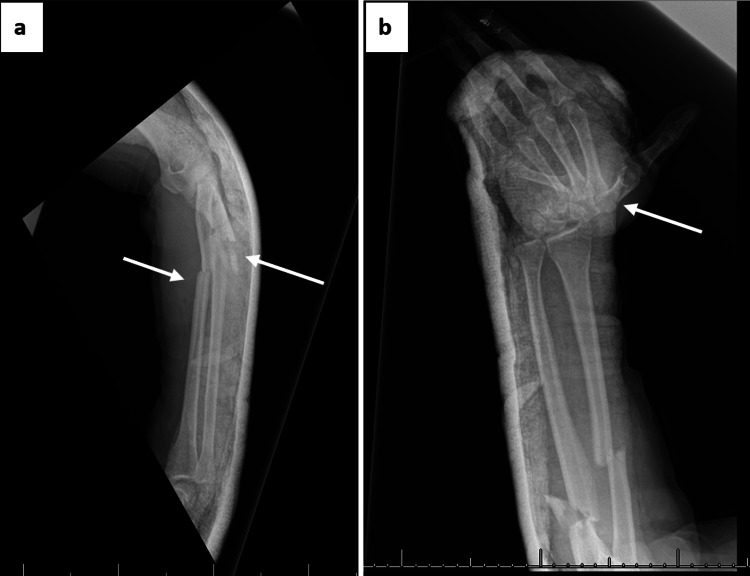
Initial radiographs: (a) lateral view showing comminuted fractures of the radius and ulna, (b) anteroposterior view demonstrating ulnar styloid fracture and wrist dislocation.
